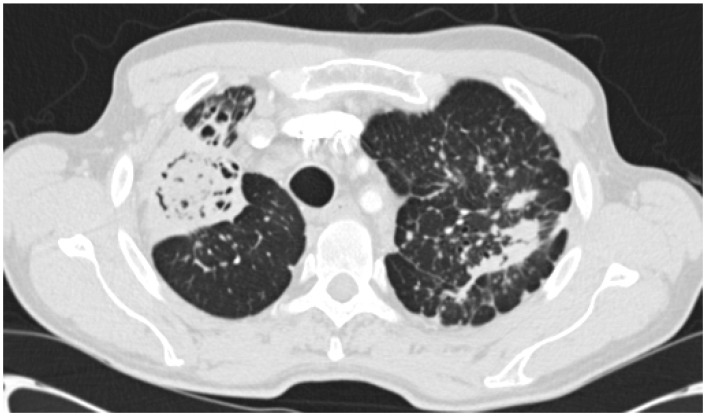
CT scan of SAIA demonstrating right upper lobe aspergilloma with associated pleural thickening and left upper lobe consolidation.
